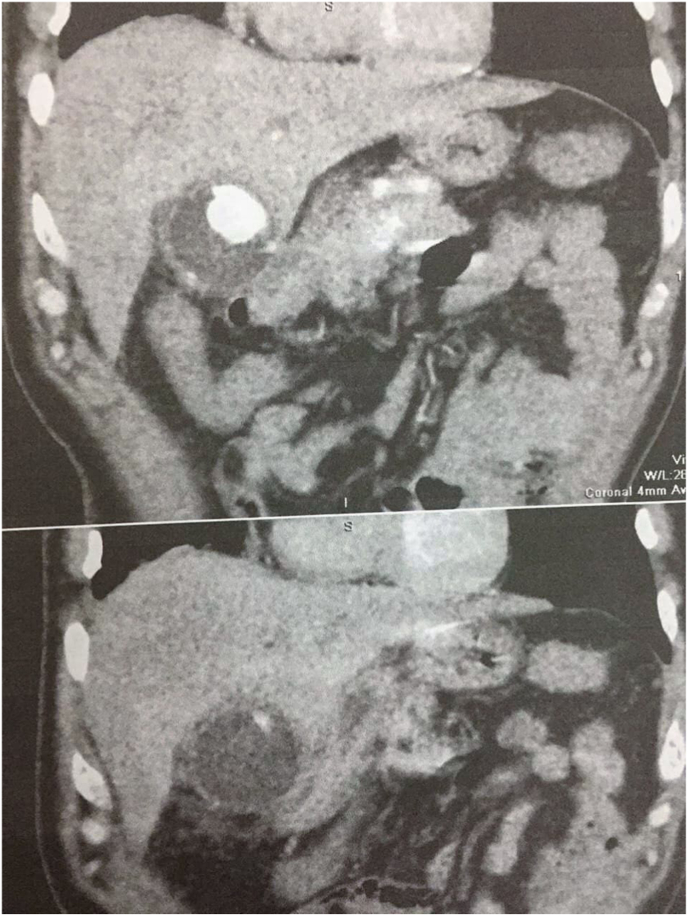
CT: Dilated gallbladder measuring 5 × 10 cm with primary hydatid cyst and calcified stone.
